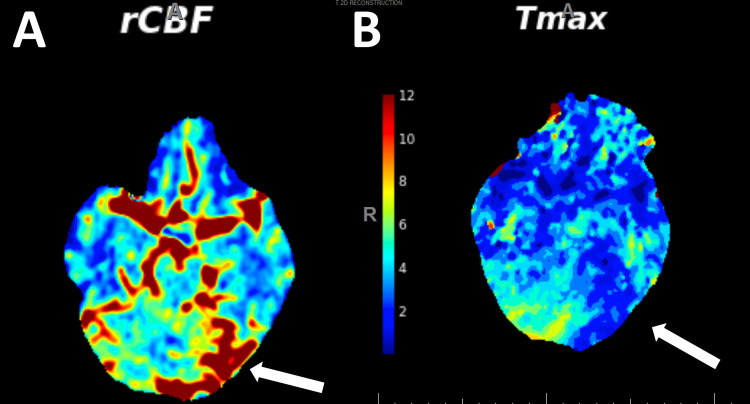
Computed tomography perfusion showing increased cerebral blood flow in the left occipital lobe (A) with correlating reduction in time-to-maximum (Tmax) (B) rCBF: regional cerebral blood flow
